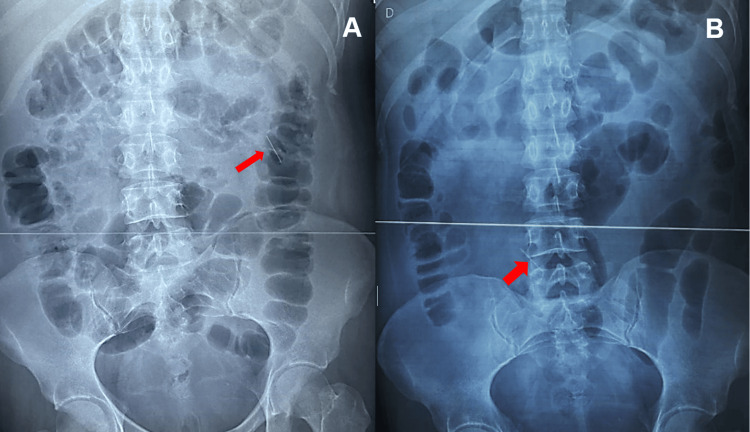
Abdominal X-ray Progression of the metallic needle in the gut (A) 12 hours after admission (B) 48 hours after admission
